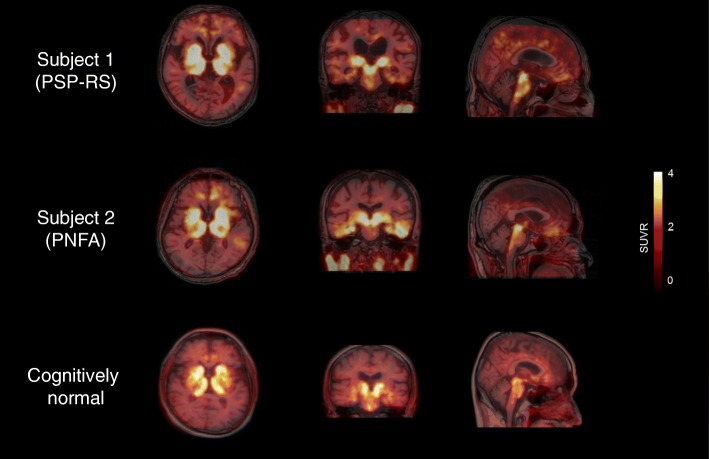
[^18^F]THK5351 PET images from two study subjects and a cognitively normal subject. The scale indicates SUVR range from 0 to 4
